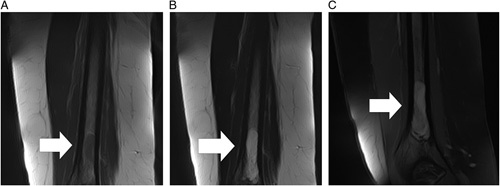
Coronal planes show an isointense to hypointense mass on unenhanced T1-weighted images (A) and isointense to hyperintense on T2-weighted images (B), with the enhancement of the vascular component on gadolinium-enhanced fat-saturated T1-weighted images (C), located in the distal third of the humerus (white arrow).
